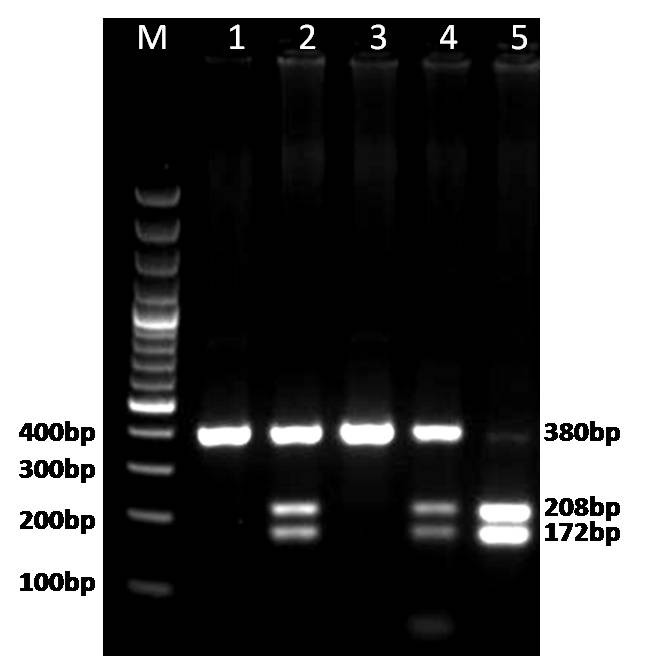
PCR-RFLP based genotyping of DNMT3B C46359T. Lanes 1 and 3: CC wild type. Lanes 2 and 4: CT heterozygotes. Lane 5: TT homozygote variant.
